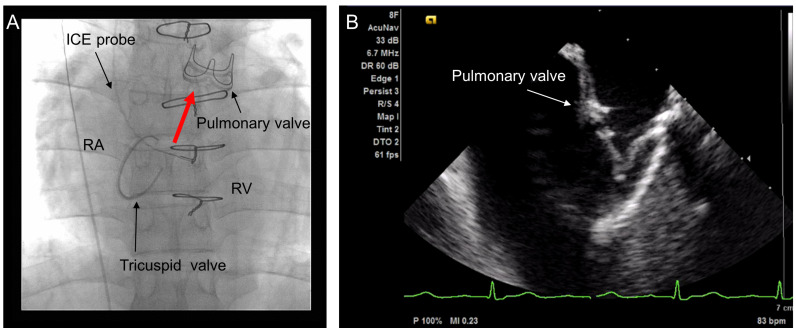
(A) Cine angiogram after pulmonary valve replacement (PVR) and (B) intracardiac echocardiography (ICE) from the internal jugular vein. A 33-year-old man diagnosed with tetralogy of Fallot underwent intracardiac repair at 6 years of age and PVR with tricuspid ring annuloplasty at 32 years of age. The ICE probe was inserted from the internal jugular vein. The red arrow shows the echo beam. ICE shows excellent visualization of the prosthetic pulmonary valve. RA, right atrium; RV, right ventricle.
